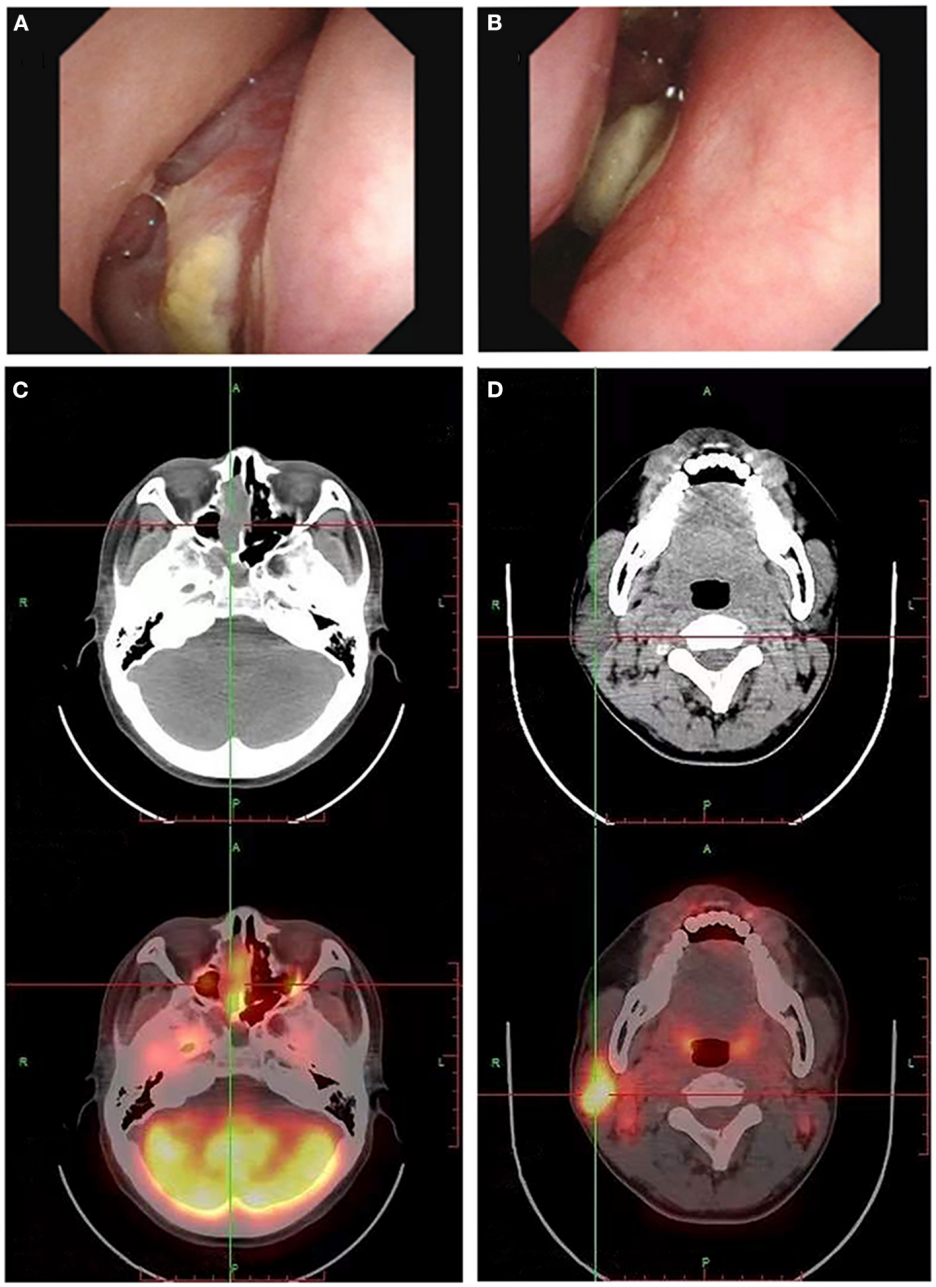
Electronic nasopharyngoscopy reveals a neoplasm in the right nasal cavity (A, B). The fluorodeoxyglucose PET/CT scan may show multiple high metabolic signals in the nasal cavity (C) and cervical lymph nodes (D), suggesting the nasal cavity neoplasm growth and lymph node metastasis.
